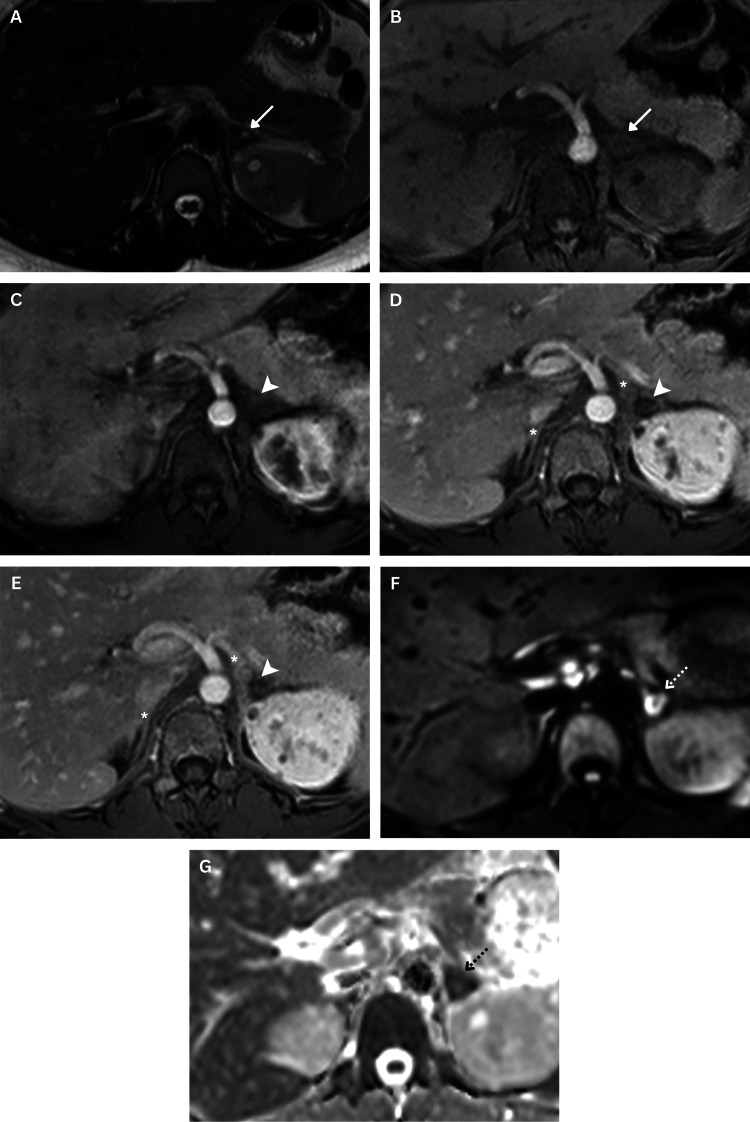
Follow-up magnetic resonance imaging. (A) Axial T2-weighted image and (B) axial pre-contrast T1-weighted image demonstrate heterogeneous signal intensity within the left adrenal gland (arrows). (C-E) Axial post-contrast T1-weighted mDixon images show a focal area of non-enhancement within the left adrenal gland (arrowheads), with preserved enhancement of the remaining left adrenal tissue and the contralateral adrenal gland (asterisks). (F) Diffusion-weighted imaging demonstrates high signal intensity with corresponding low signal on the apparent diffusion coefficient map (G), consistent with diffusion restriction and supporting the diagnosis of adrenal infarction with hemorrhagic transformation.
